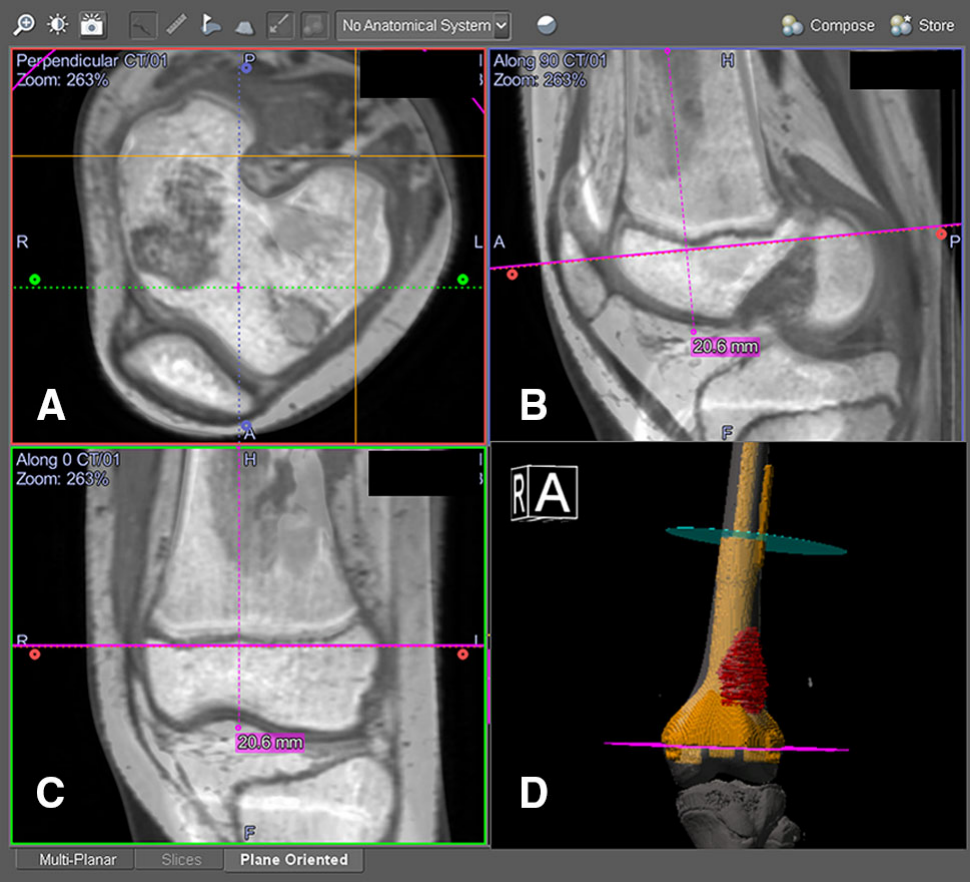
CT/MR fusion images are shown in the navigation display in a 13-year-old girl with a left distal femoral osteosarcoma. Joint-preserving resection and reconstruction were performed with a custom CAD prosthesis. The intraosseous tumor extent was better seen on the T1-weighted MR images. The resection plane (*pink*) was defined as 2 cm proximal to the knee joint. The resection level could be checked with the axial (a), reformatted sagittal (b), and coronal (c) views of the fused images. CAD data of the joint-preserving prosthesis also could be imported into the navigation planning and seen on the 3D model (d) with the resection planes. Surgeons checked the final design of the CAD prosthesis before giving approval to the manufacturer
